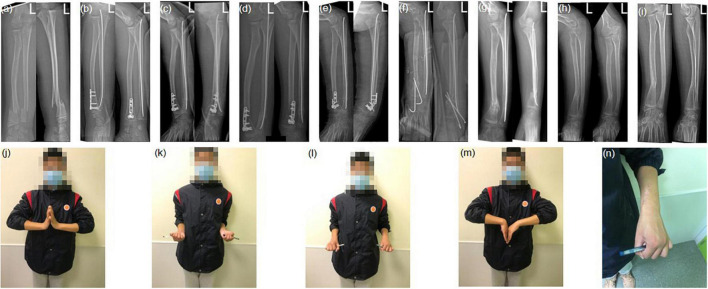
A male patient, 11 years and 9 months old, with fracture of the left radius and ulna. Open reduction internal fixation with one plate and five screws was used to treat the radius fracture and closed antegrade intramedullary nail fixation (2.0 mm diameter) to treat the ulna fracture. Anteroposterior and lateral radiographs: (a) preoperative; (b) 1 day postoperative; (c) 6 weeks postoperative; (d) 10 weeks postoperative (delayed union); (e) 3 months postoperative (plate fracture); (f) 3 months postoperative (reoperation performed with two 2.0-mm-diameter Kirschner wires fixation and iliac crest bone graft); (g) 5.5 months postoperative (2.5 months after the reoperation and after removal of the two Kirschner wires); (h) 13 months postoperative (after removal of one plate); (i) 16 months postoperative (final follow-up). Final follow-up: (j) pronation; (k) supination; (l) no limitation of dorsiflexion; (m) there is 20° limitation of palmar flexion; (n) scar.
